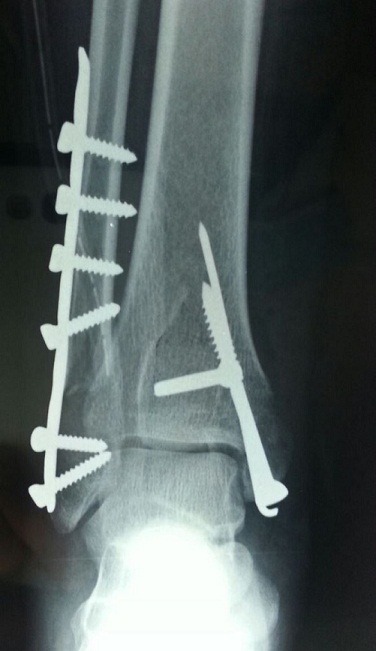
Radiographie de face cheville gauche montrant le contrôle post opératoire
